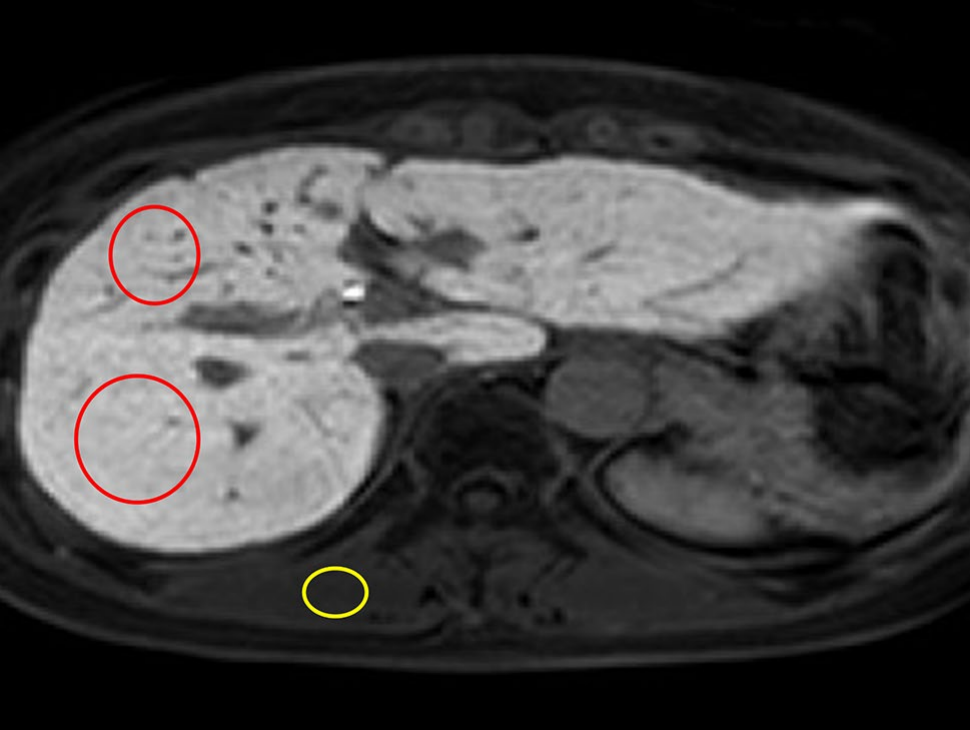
The hepatobiliary phase of gadoxetic acid-enhanced MRI. The signal intensities were measured by placing two round or oval regions of interest (ROIs) (red circles) on the remnant liver parenchyma, and one round or oval ROI on the erector spinae muscle (yellow circle) as large as possible
